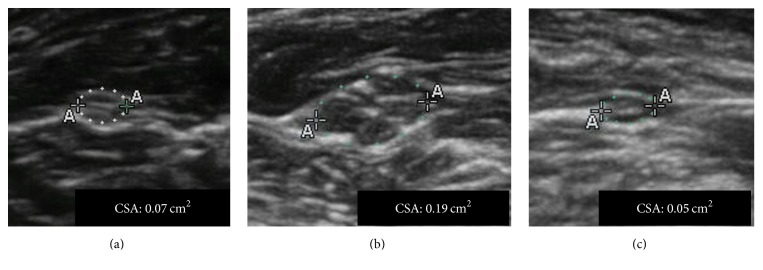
Median nerve axial ultrasound scan in CIDP patients at different disability state. (a) Male, 60 years old; disease duration 64 months; INCAT upper limbs score: 1. (b) Male, 63 years old; disease duration 72 months; INCAT upper limbs score: 2. (c) Male, 62 years old; disease duration 79 months; INCAT upper limbs score: 4.
